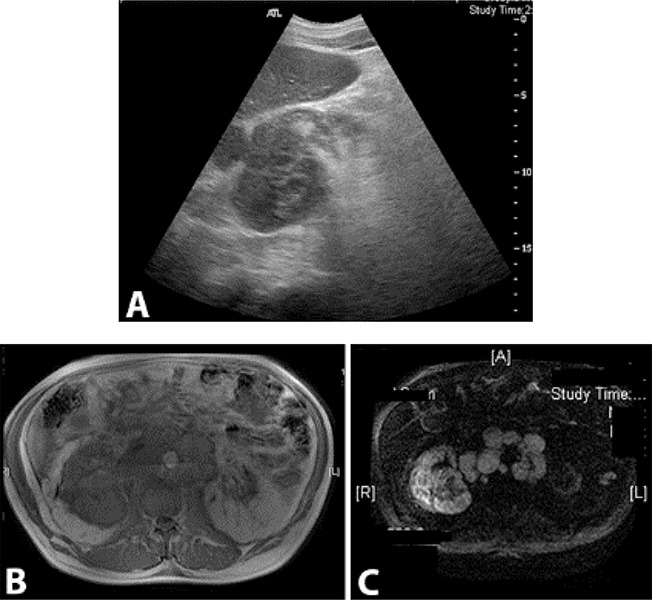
A, Renal ultrasound revealed multiple hypoechoic lesions in the right native kidney. Abdominal MRI showed an enlarged right native kidney with irregular margins (B). Additionally, retroperitoneal adenopathy was observed (C).
